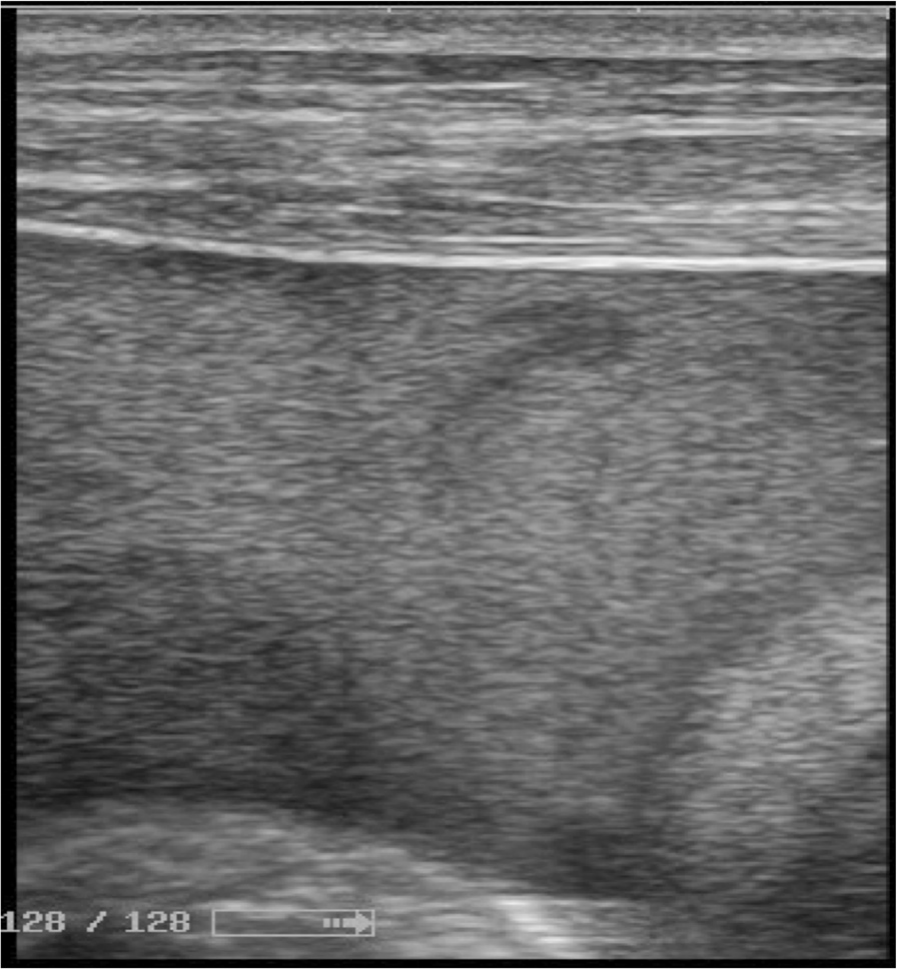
Ultrasound image of an intraperitoneal effusion containing multiple mobile echoes emphasizing its hematic origin
